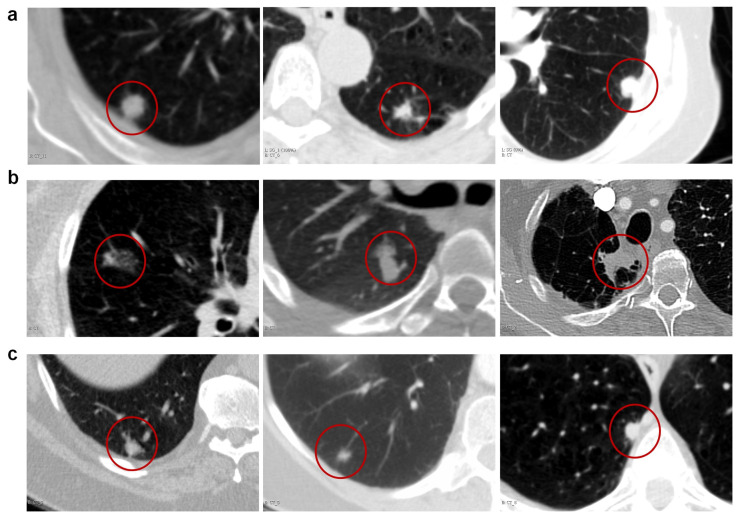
Examples of difficult cases that misclassified by readers. (a) Axial CT images of biopsy proven three pulmonary adenocarcinomas that were misclassified by both readers and correctly classified by machine (NIS classifier). (b) Three pulmonary granulomas that were misclassified by both readers and correctly classified by machine. (c) Three pulmonary adenocarcinomas that were misclassified by both human and machine.
